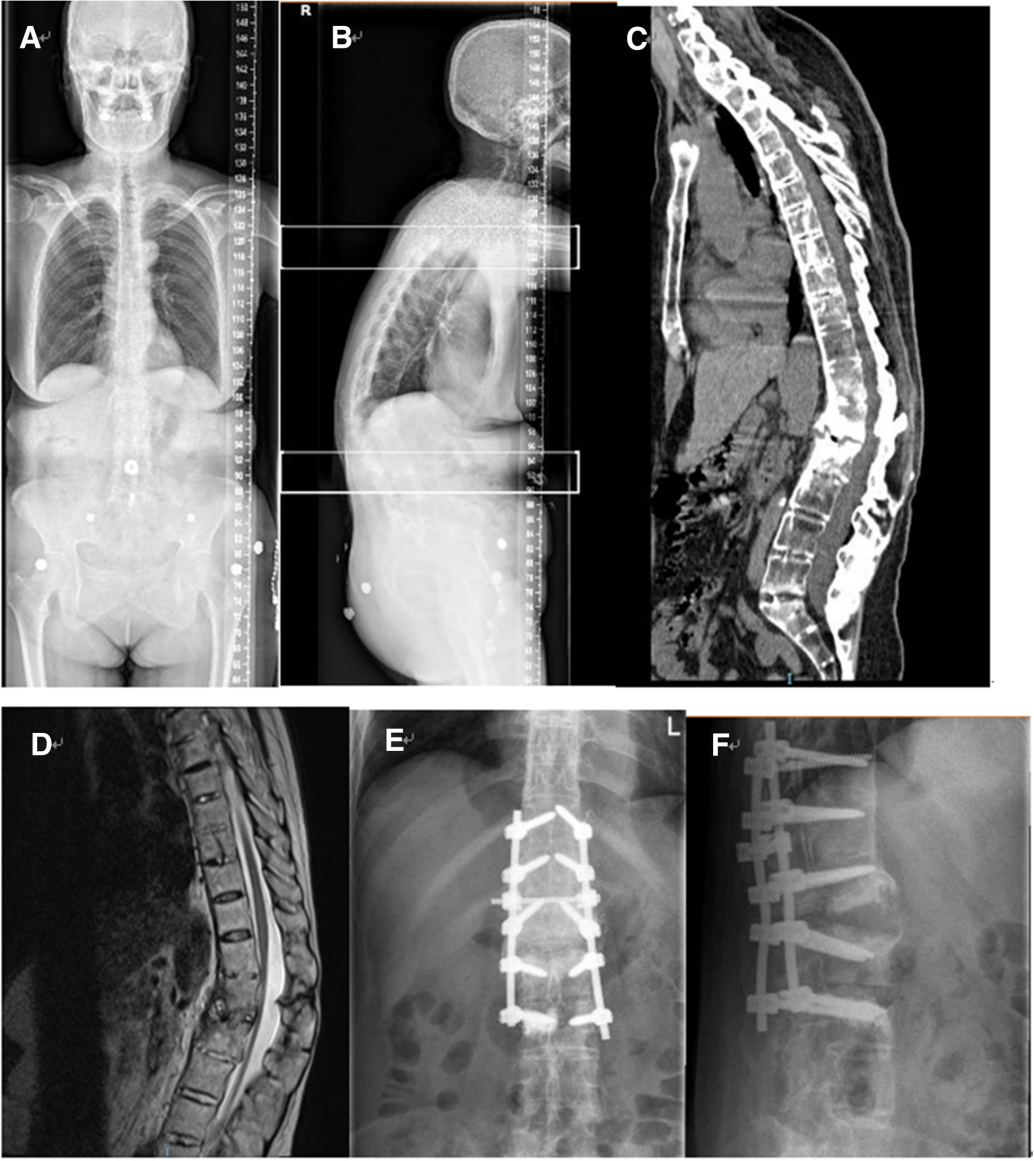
This patient had a history of AS for more than 36 years, with severe kyphosis. He was admitted to the hospital due to low back pain, without any obvious trauma. There was no spinal cord injury before surgery. a-b Preoperative X-ray shows a severe kyphosis. c Preoperative CT shows obvious vertebral calcification and osteoporosis, the fracture position was L1/2. d Preoperative MRI shows significant signal changes between L1/2. e-f Postoperative X-ray shows satisfactory pedicle screw position and good resetting effect
